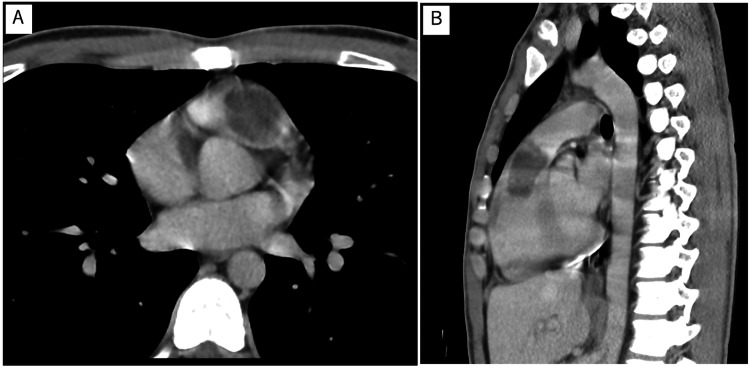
CT images of the tumour in the right ventricular outflow tract Axial (A) and sagittal (B) views of the tumor in the right ventricular outflow tract on CT. CT: computed tomography
